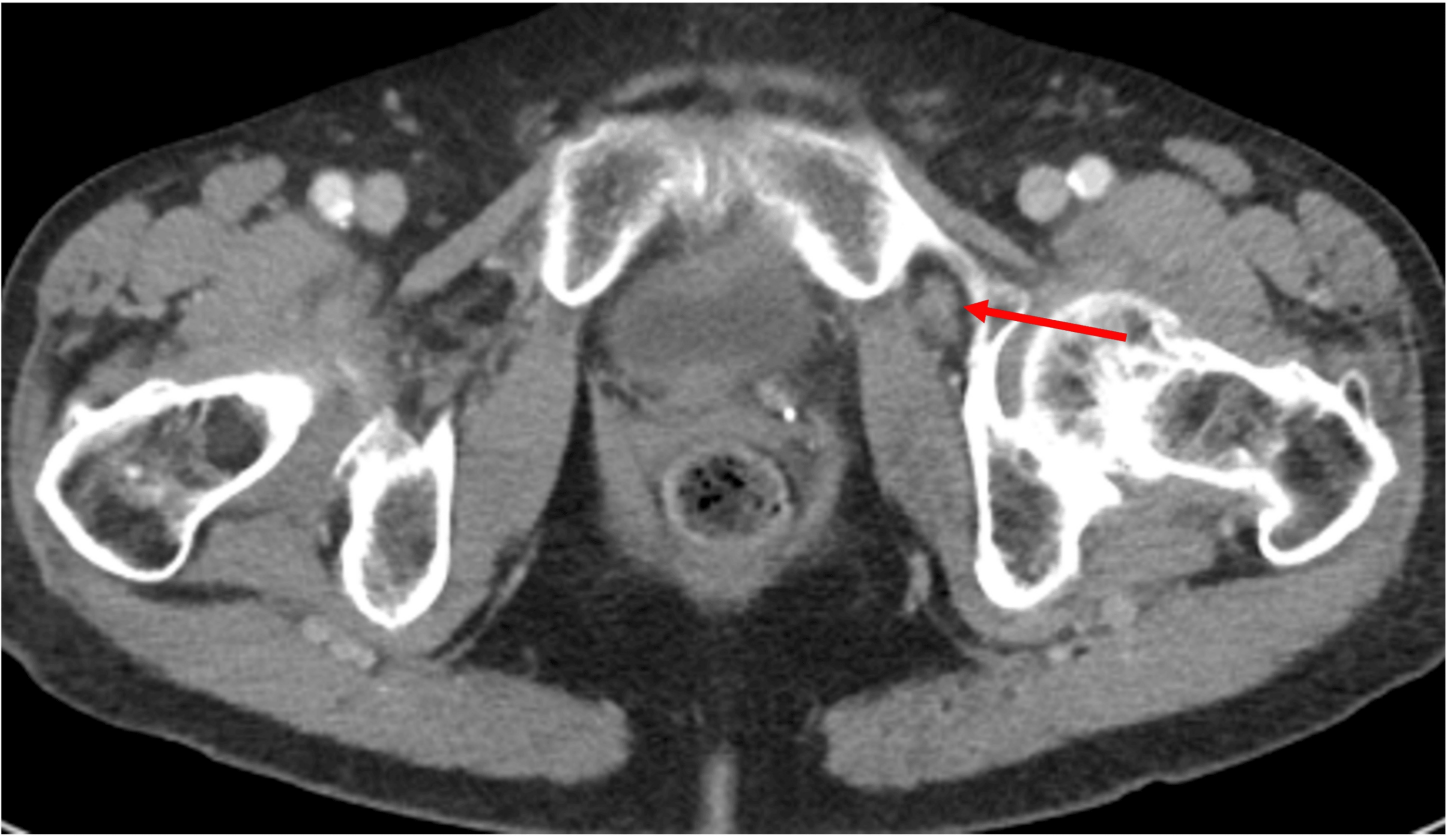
Contrast-enhanced axial computed tomography image demonstrating the decompressed bladder diverticula within the obturator foramen.
